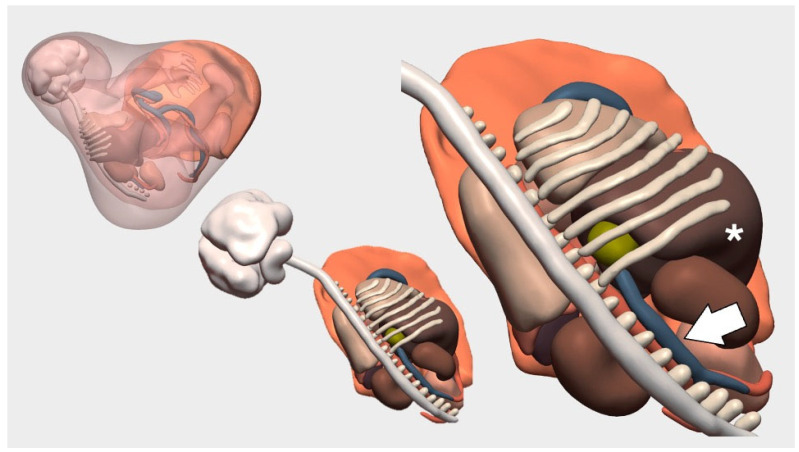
Virtual 3D reconstruction of right-sided diaphragmatic hernia based on fetal MRI. The different fetal planes are visualized and can be selectively removed in the Surgical Planner to better assess the dimensions of the diaphragmatic hernia. The liver is marked with an asterisk, and next to it, the infrahepatic vena cava can be seen (white arrow).
